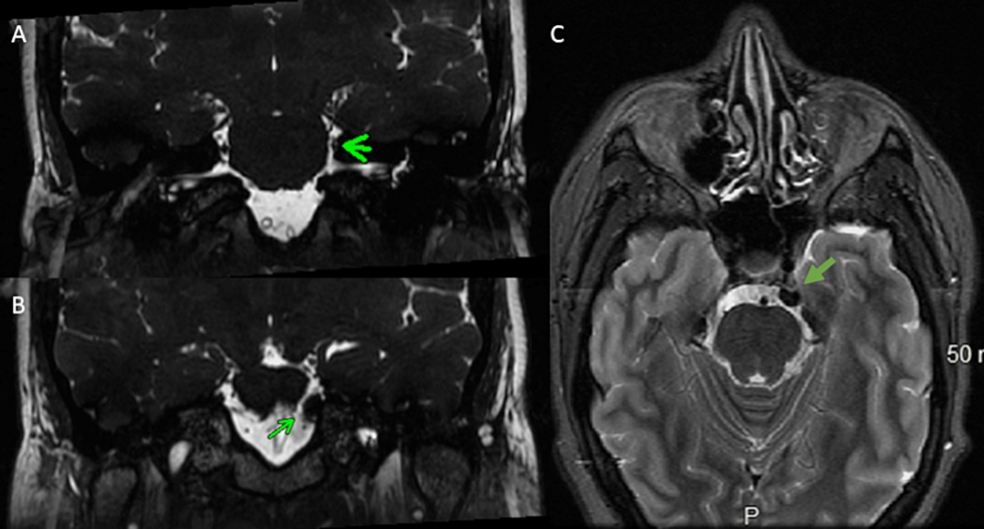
Preoperative brain MRI, coronal view (A and B) and axial view (C) The view shows osteomatous growths along the left petrous bone compressing the trigeminal nerve MRI: magnetic resonance imaging
